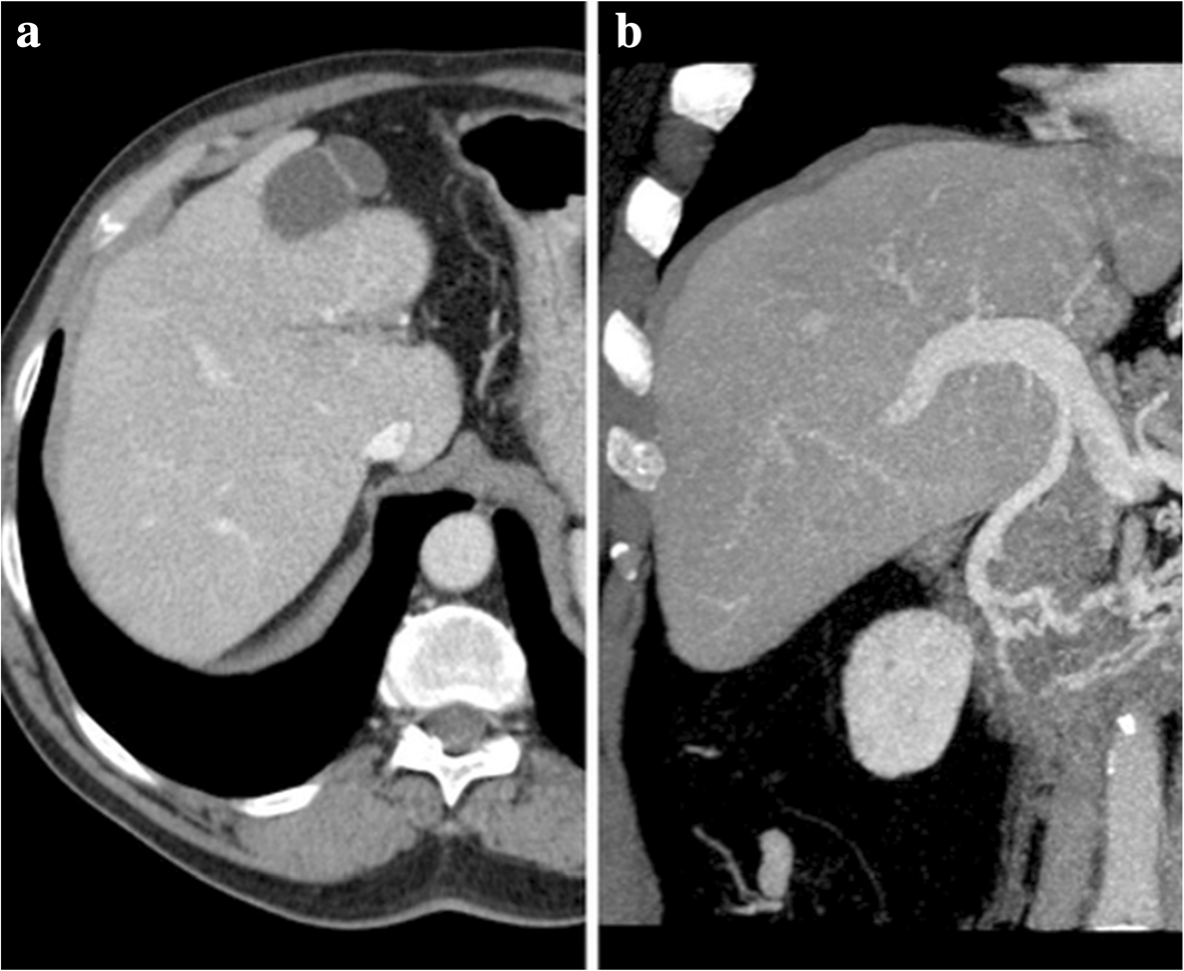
Portal vein thrombosis, chronic evolution. a MPR and b MIP images from portal venous phase CT show the evolution of a previous left PV complete thrombosis. With time, the unsupplied hepatic segments totally disappeared with atrophic involution of the left lobe
